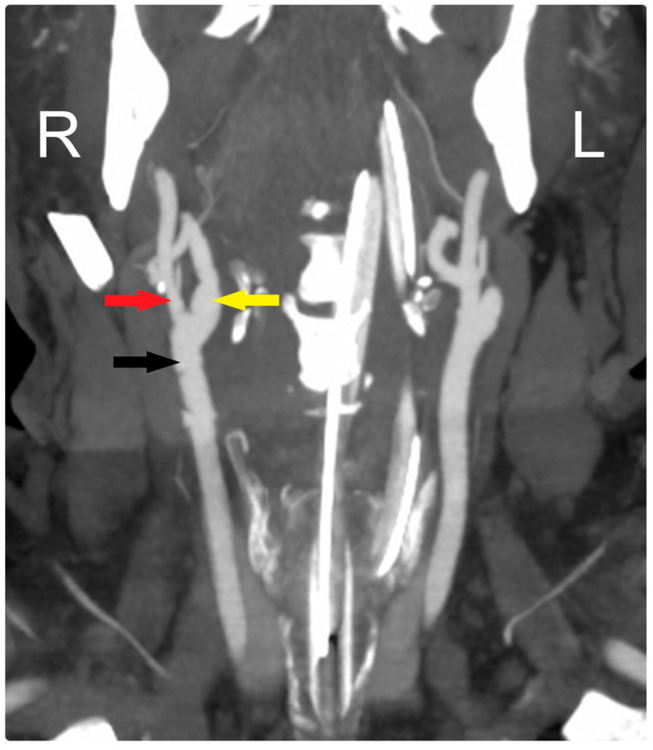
Early postoperative CTA shows patency of all arteries: CCA (black arrow), ECA (red arrow), and ICA (yellow arrow). R: Right, L: Left.
